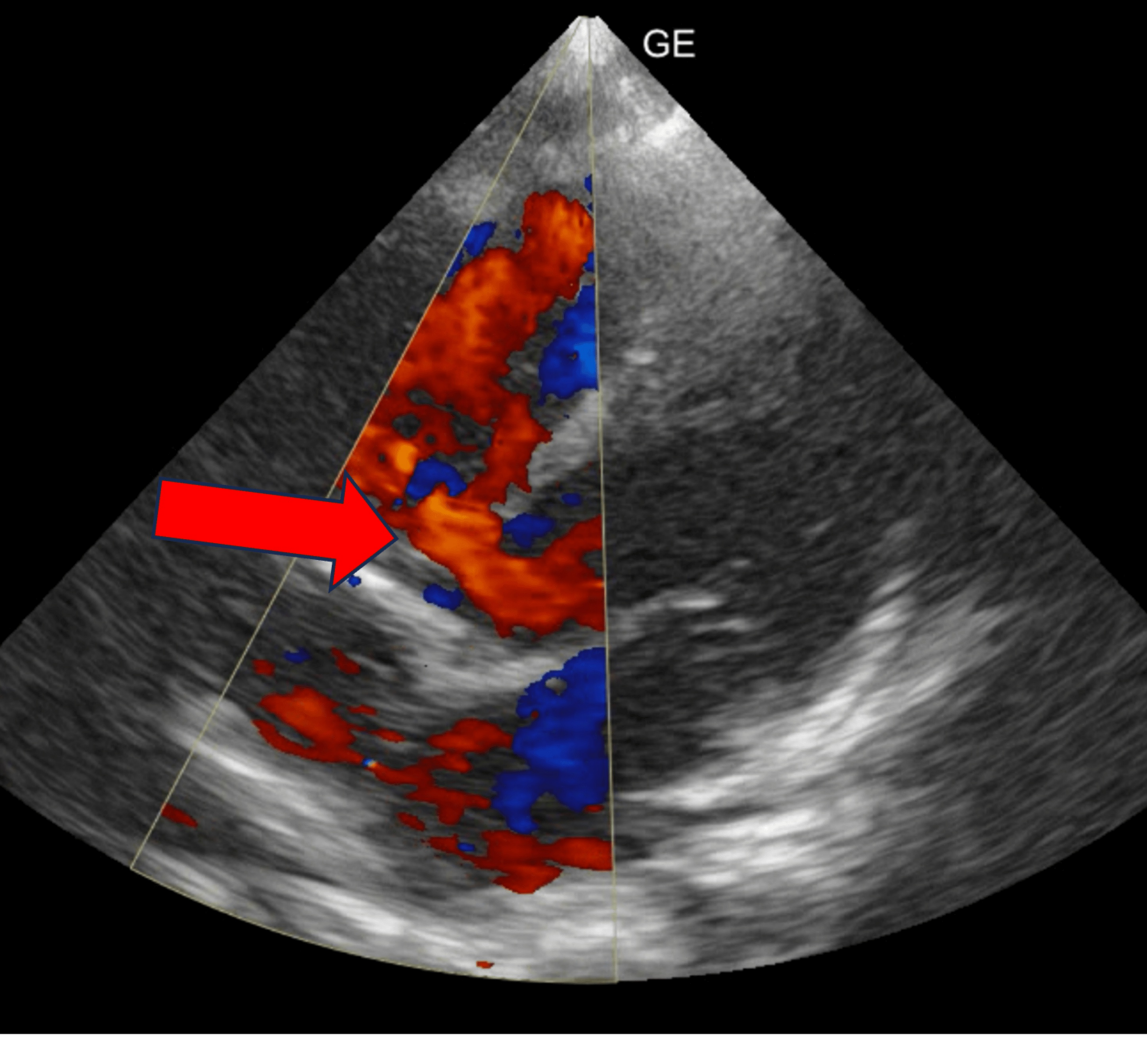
A 2D echocardiography revealed ventricular septal defect (red arrow).
